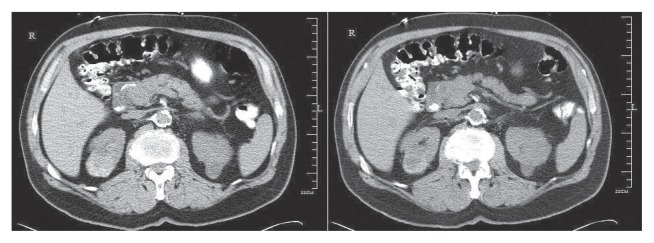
CT images of the abdomen with contrast medium shows a heterogeneously enhancing exophytic mass projecting posteriorly from the upper pole of the right kidney.
